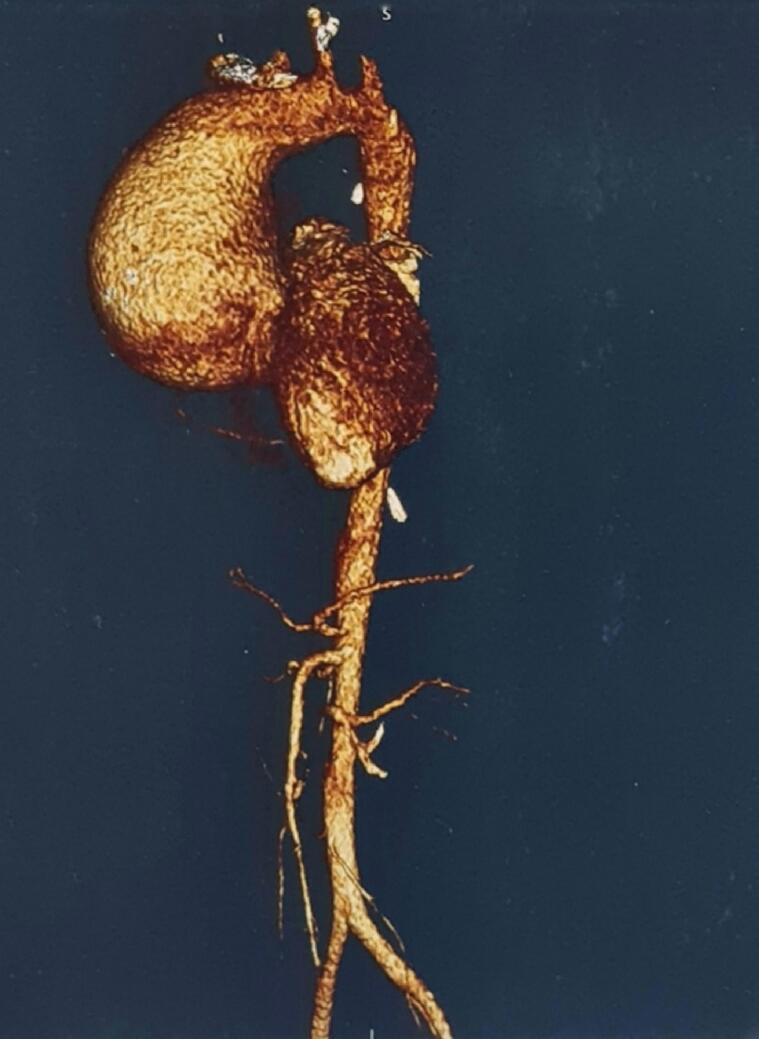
3D reconstruction CT angiography. Marked dilatation of the ascending aorta is noted.
